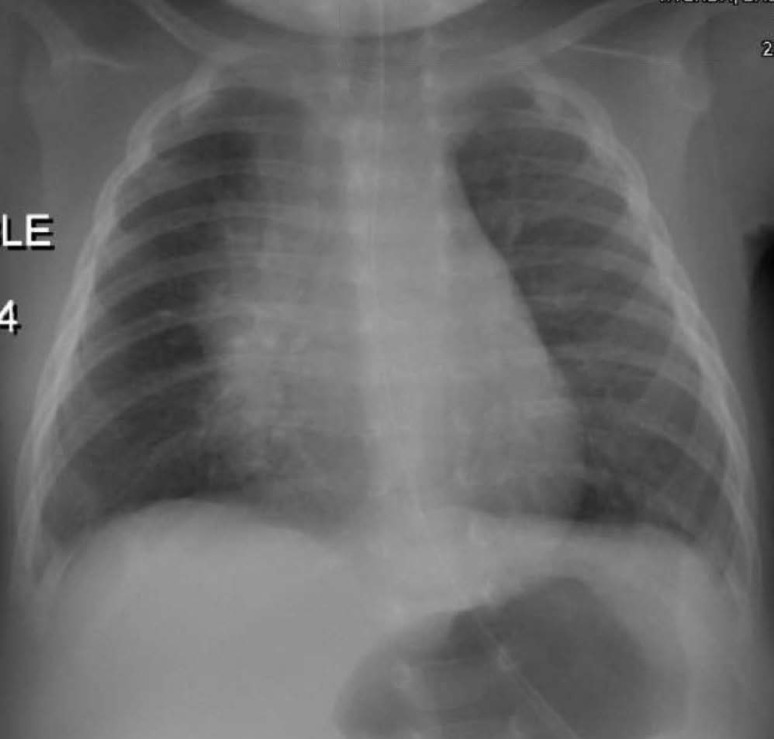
Chest X-ray was performed following the admission of the third case to the PICU. In comparison to the previous chest X-ray, there is a progression of retrocardiac and left lower lung zone infiltrations.
